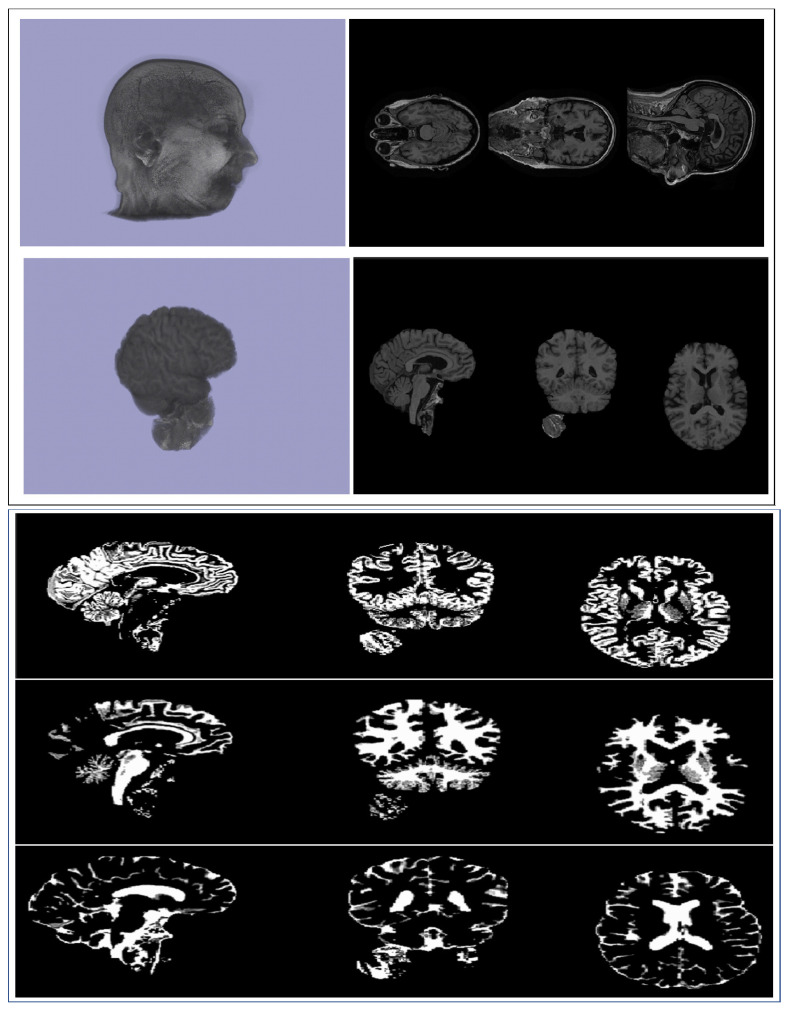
(Top row) An original MRI image and the corresponding axial views. (Second row) Brain image extracted using BET tool and its axial views. (Bottom three rows) Segmentation of the extracted brain image using FAST; from top to bottom: gray matter (GM), white matter (WM), and cerebrospinal fluid (CSF) images, respectively.
